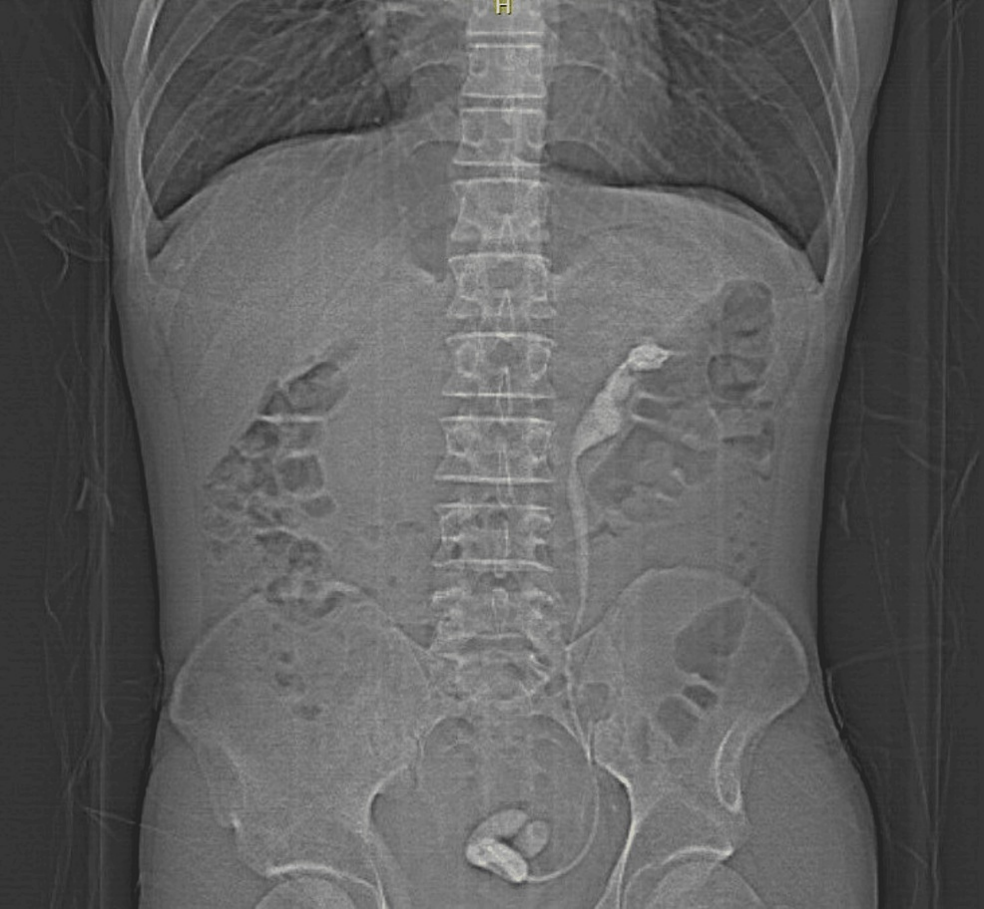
Abdominal X-ray image revealing calcification throughout the entire trajectory of double J stent (renal pelvis, ureter, and urinary bladder).
